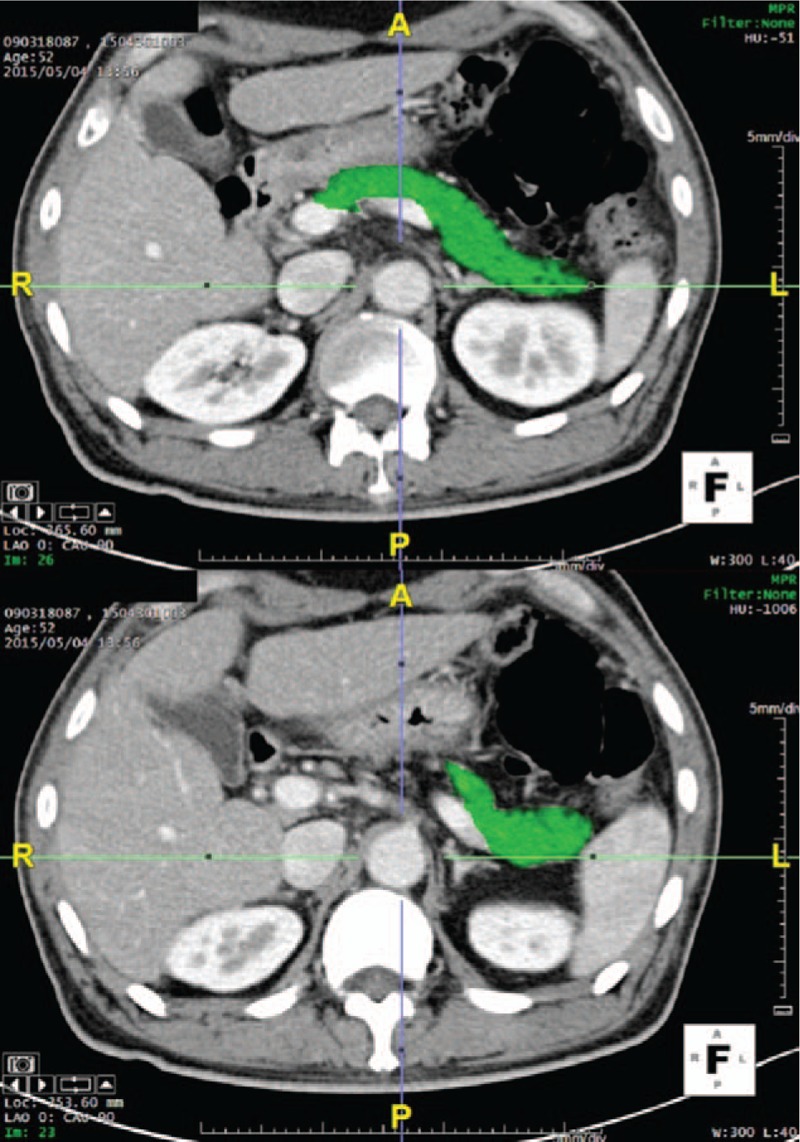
CT images of pancreatic phase were transported from PACS (Picture Archiving and Communication System) to the TeraRecon (San Mateo, CA) server. We drew the border of the pancreas by using the free-ROI manual method in the axial image.
